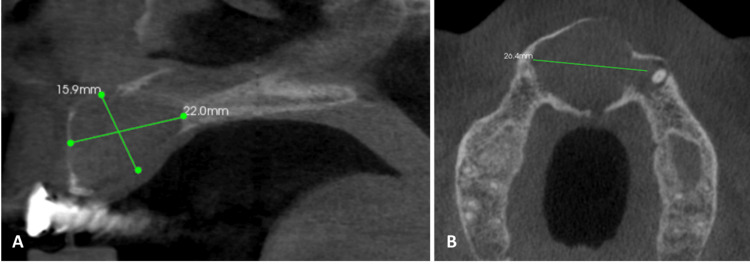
Cone-beam computed tomography of the case showing the nasopalatine duct cyst (NPDC) in sagittal (A) and axial (B) cuts
